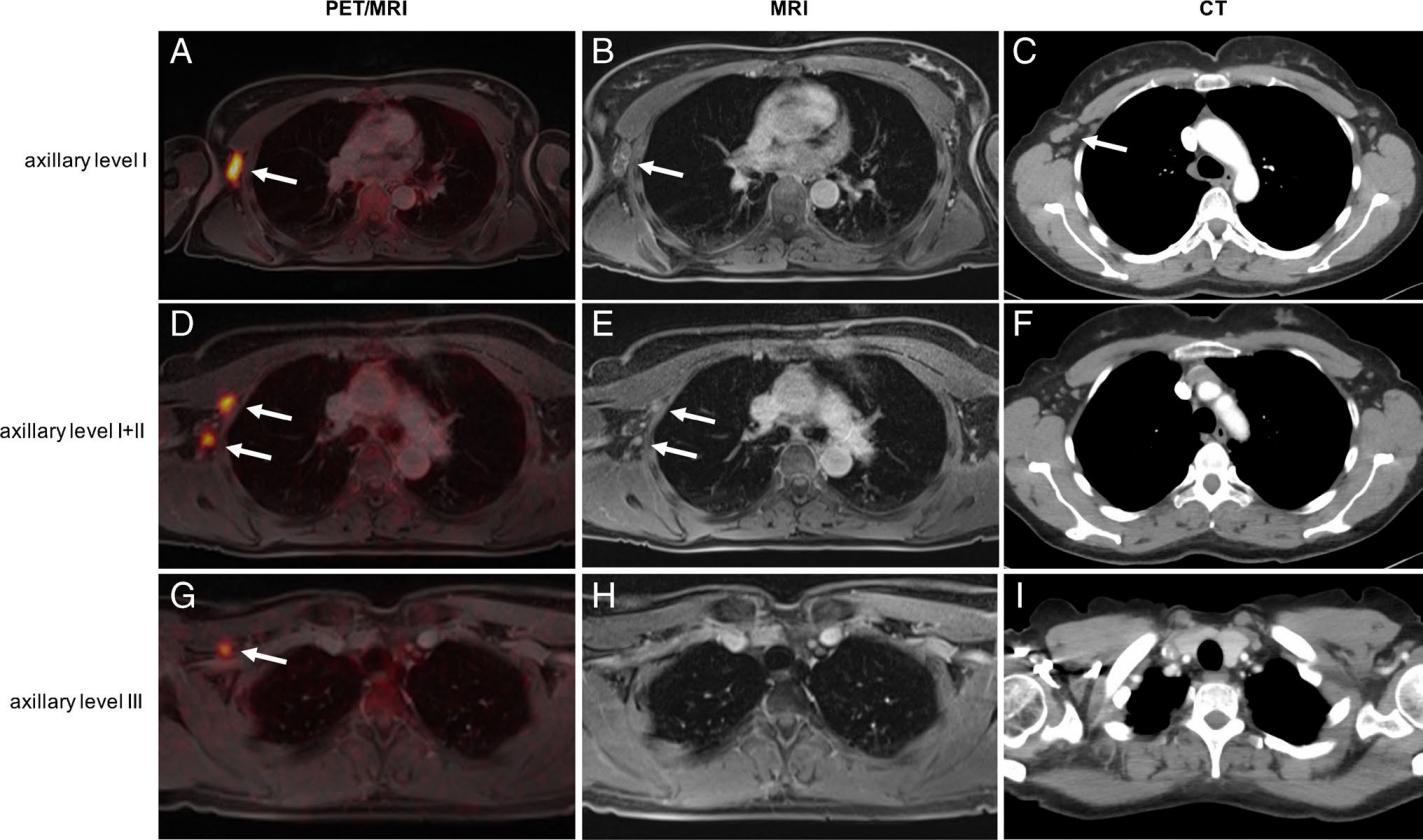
Lymph node metastases (white arrows) detected in different imaging modalities. A–C Lymph node metastasis in axillary level I detected in PET/MRI (A), MRI (B), and CT (C). D–F Lymph node metastases in axillary levels I and II detected in PET/MRI (D) and MRI (E), but not in CT (F). G–I Lymph node metastasis in axillary level III detected in PET/MRI (G), but not in MRI (H) and CT (I)
